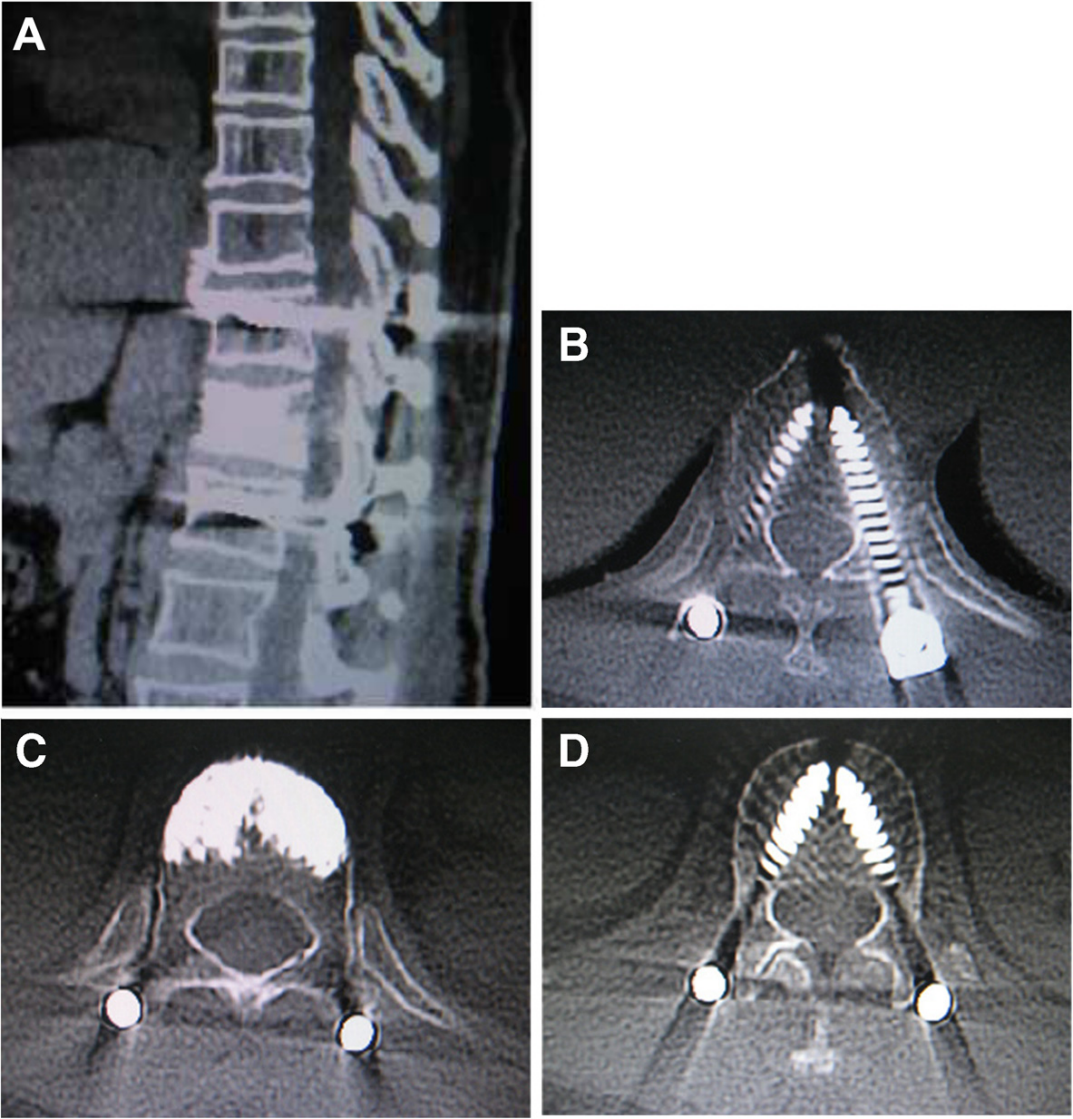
Postoperative CT scanographic images. Sagittal (A) and axial CT-scan (B), (C), (D) immediate postoperative reconstruction, verification of pedicle screws positioning and search for cement leakage.
